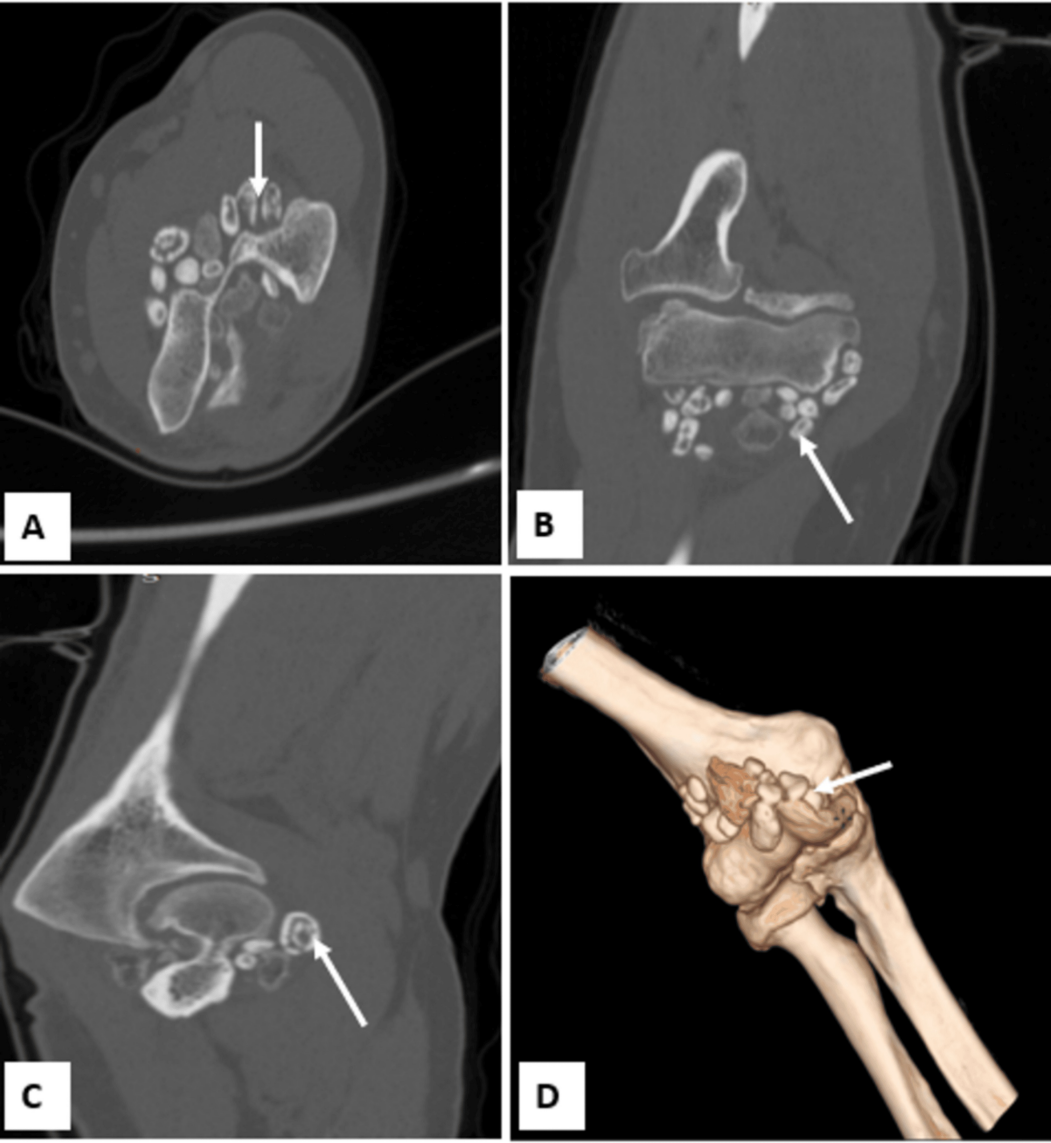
CT scan of the elbow (A) axial plane, (B) coronal plane, (C) sagittal plane, (D) three-dimensional reconstruction Showed multiple calcifications of the periarticular soft tissues(white arrow), associated with sclerotic lesions and pinching of the joint space.
